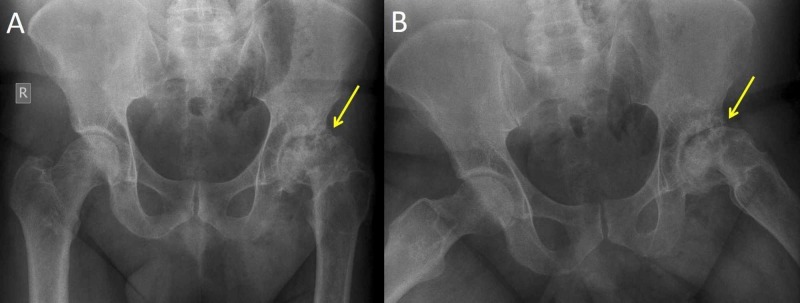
Marked osteoarthritis of the left hip secondary to non-diagnosed SCFE in a 40-year-old man The x-ray was taken while his 11 year-old-daughter was treated for bilateral SCFE. Anteroposterior (A) and frog-lateral (B) pelvis views indicate marked osteoarthritis secondary to untreated SCFE of the left hip (arrows). SCFE: slipped capital femoral epiphysis
